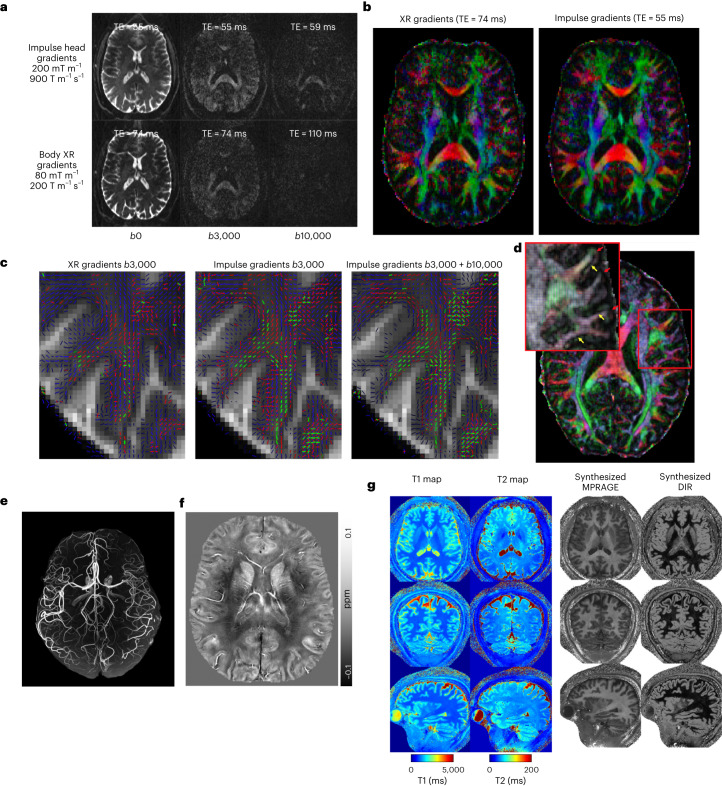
Diffusion and structural imaging. a, Diffusion-weighted MRI at various *b* values. Comparing images acquired with NexGen 7 T gradient coil performance in the top row to conventional gradient coil performance (80 mT m^−1^, 200 T m^−1^ s^−1^) in the bottom row. b, Improvement in color principal fiber orientation maps with shorter TE and higher SNR using NexGen 7 T gradient coil performance. c, Improvement in crossing fiber detection in complex white matter regions: primary (blue), secondary (red) and tertiary (green) fiber crossings in centrum semiovale white matter. The vector color intensities are modulated by the fiber’s respective volume fraction. Only fibers with volume fractions greater than 5% are shown. d, Pushing the spatial resolution to 0.8 mm using NexGen 7 T gradient coil. The primary diffusion direction map is overlaid onto fractional anisotropy for this 0.8 mm data. Yellow arrows indicate where white matter tracts turn sharply into the cortex and red arrows denote gyral crowns where the white matter tracts continue straight into the gray matter. Adapted from ref. ^60^. e, Time-of-flight 3D MRA at 0.4 mm isotropic. f, 3D QSM with tenfold acceleration. QSM map of a representative axial slice with a resolution of 0.21 × 0.21 × 1.5 mm^3^, reconstructed using the software STI Suite (UC Berkeley). g, Whole-brain quantitative mapping and synthesized images using MR fingerprint spiral imaging provided multiple image contrasts at 0.56 mm isotropic in a 4-min acquisition time. From left to right: acquired whole-brain T1 and T2 maps and several derived 3D image sets with different contrasts. MPRAGE (magnetization prepared-rapid GRE), double inversion recovery (DIR).
